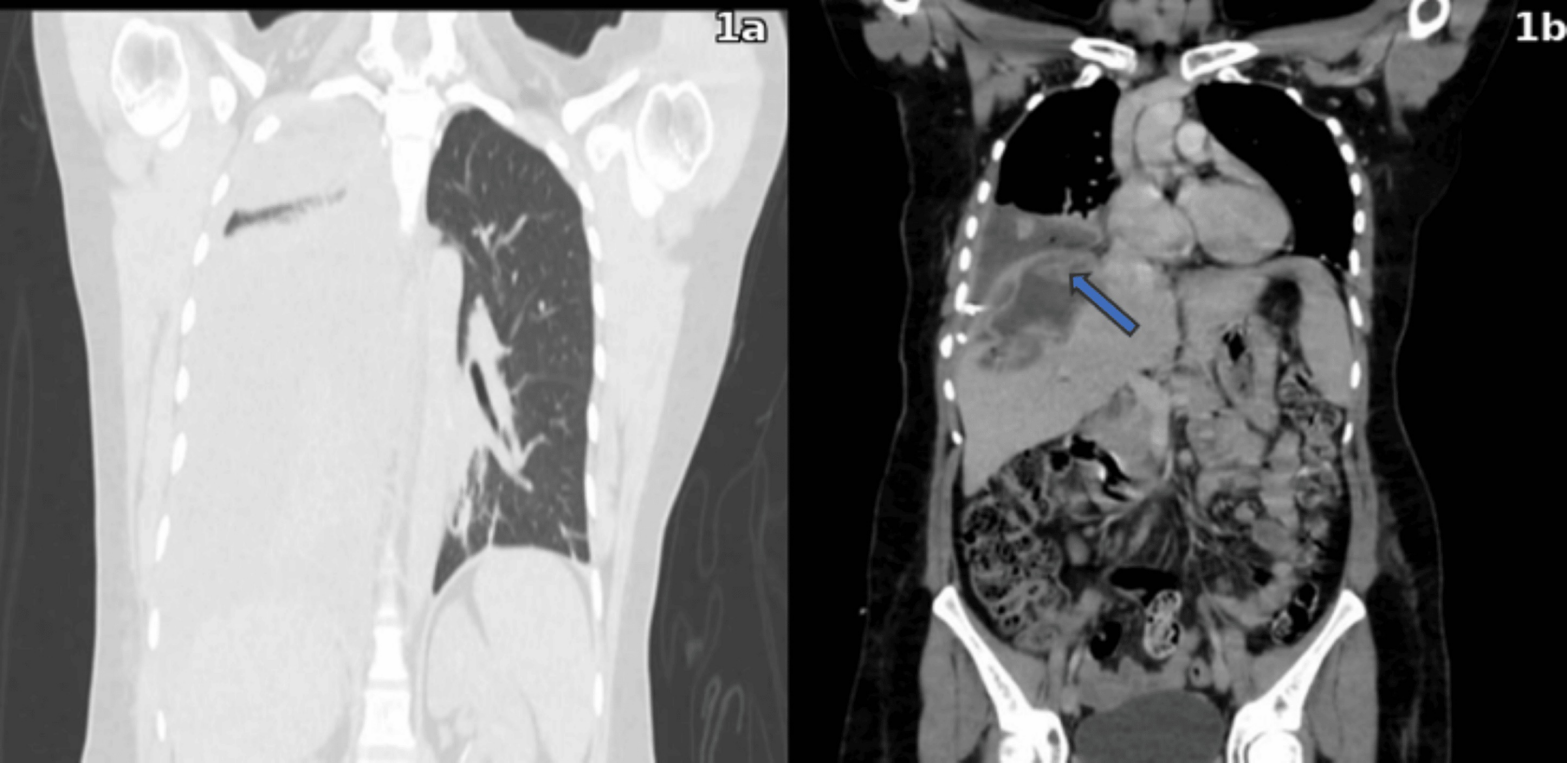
Contrast-enhanced chest CT 1a. Coronal section showing a right pleural effusion; 1b. Coronal section demonstrating a communication between the diaphragm and the right hepatic lobe, suggestive of a hepatopleural fistula (blue arrow).
